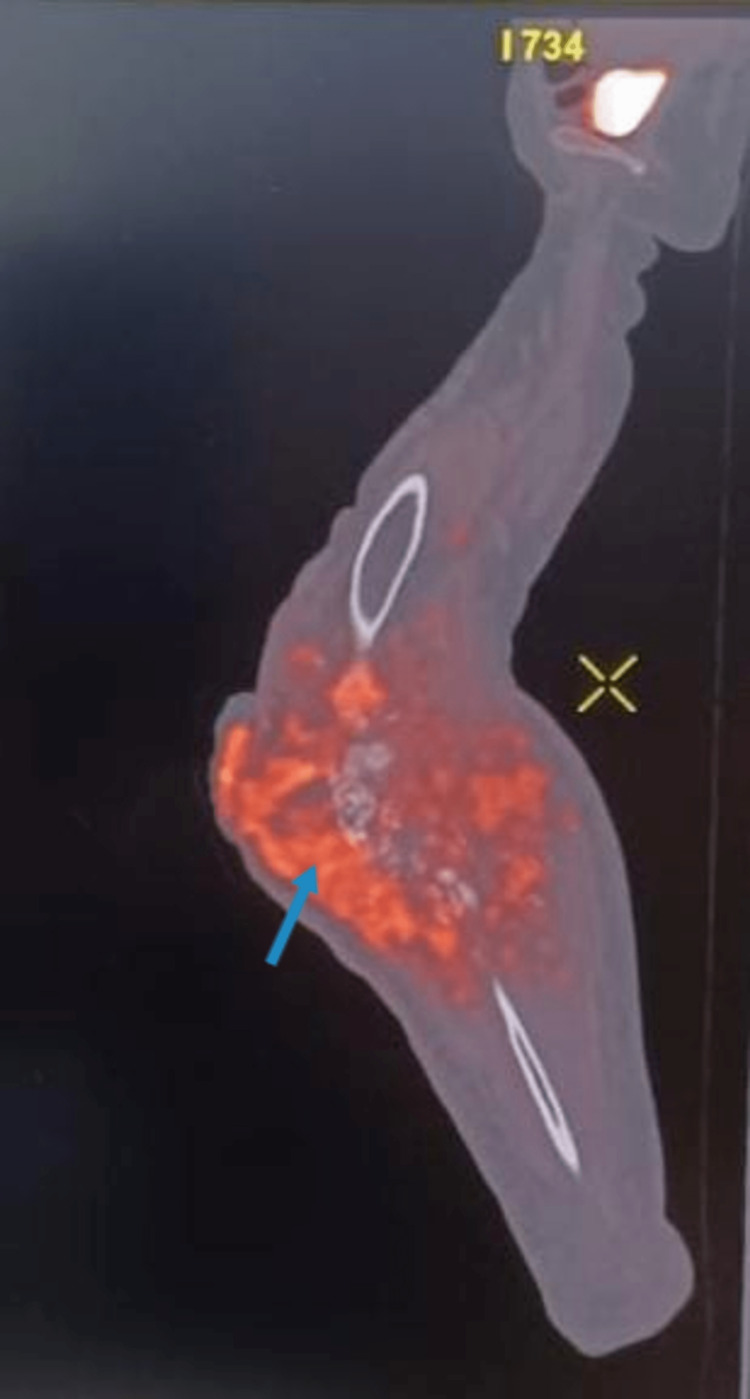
Positron emission tomography-computed tomography showing enhanced tumour lesions with involved soft tissue around proximal tibia and fibula (blue arrow). Figure credits: Harshika Kaur Saluja (author).
